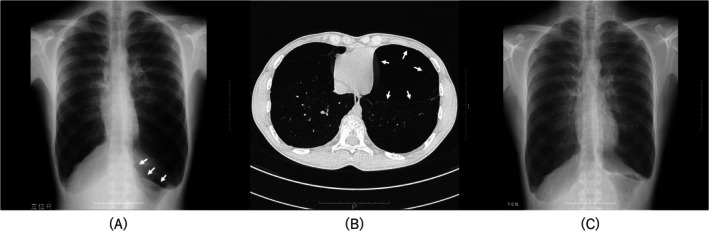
(A) Preoperative chest radiography showing bilateral lung hyperinflation and the depression of the left diaphragm (arrow) due to hyperinflation in the left lower lobe. (B) A chest radiography obtained 8 years postoperatively showing a physiological contour of the left diaphragm. (C) Preoperative computed tomography of the chest showing bilateral emphysematous lung parenchyma and a giant bulla in the left lower lobe.
